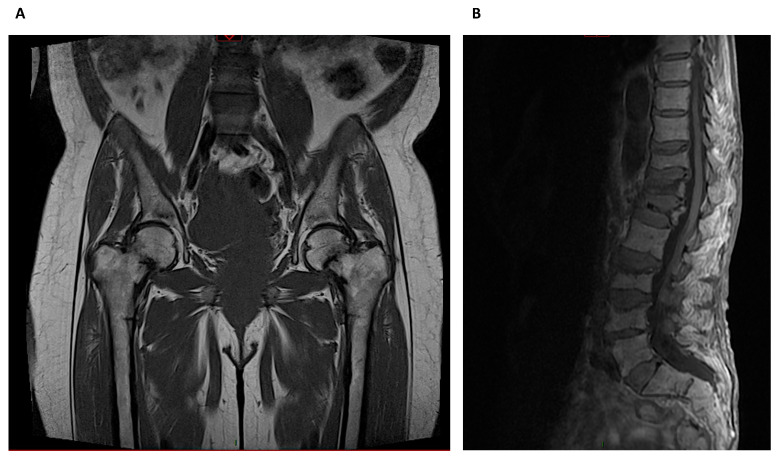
Magnetic Resonance Imaging: (A) Bilateral metaphyseal femoral fractures distal to the lesser trochanter with reactional associated bone edema in a hemodialysis patient with osteomalacia. (B) Osteoporotic lumbar spine compression fracture at T11, T12, L1, L3 and L4 vertebral body that is hyperintense on T2 and shows vivid contrast enhancement with paravertebral soft tissue edema.
